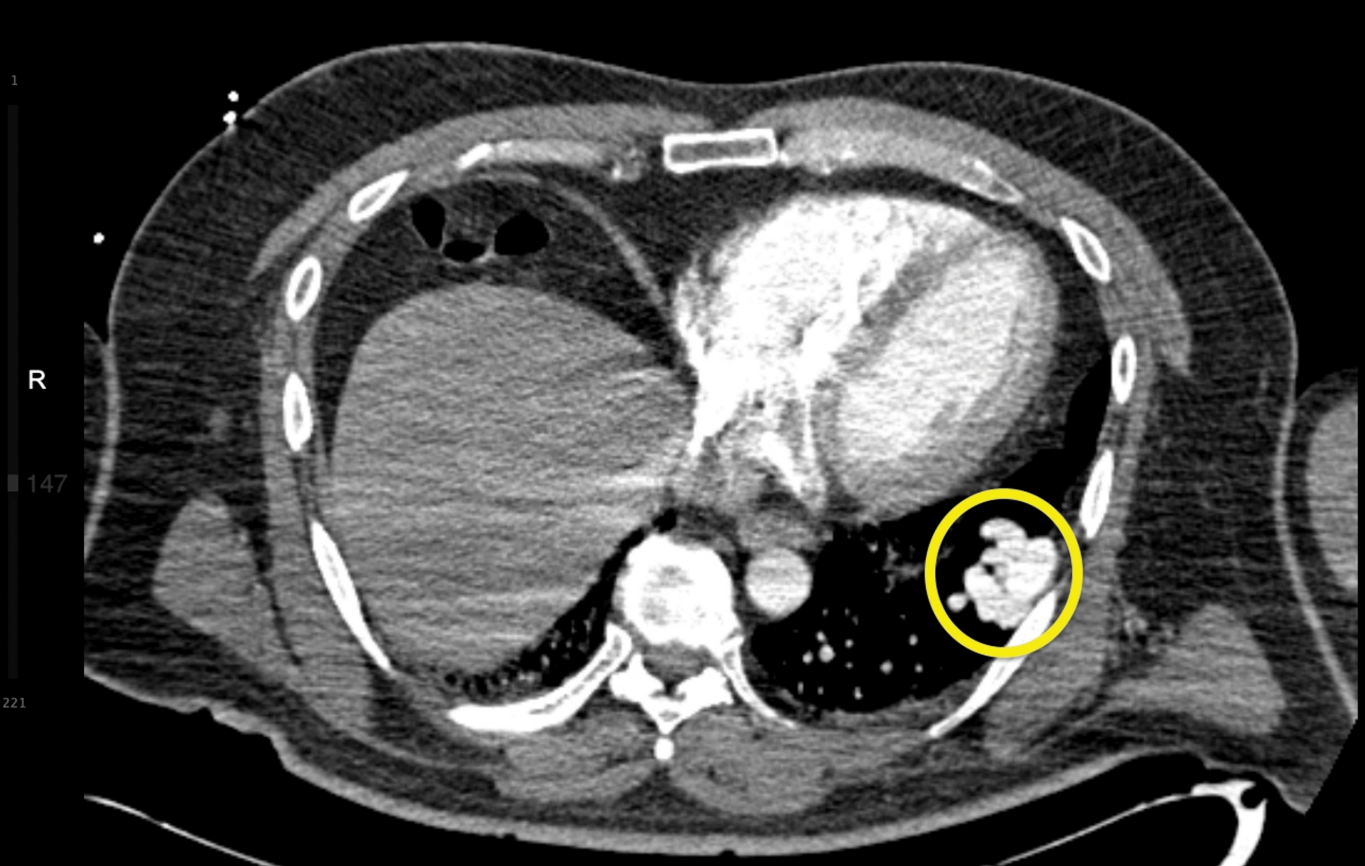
CT angiogram of chest (axial view) showing PAVM in the left lower lobe of the lung (3.5 cm x 6.8 cm) and feeding artery (1.1 cm) (yellow circle). PAVM: pulmonary arteriovenous malformation
